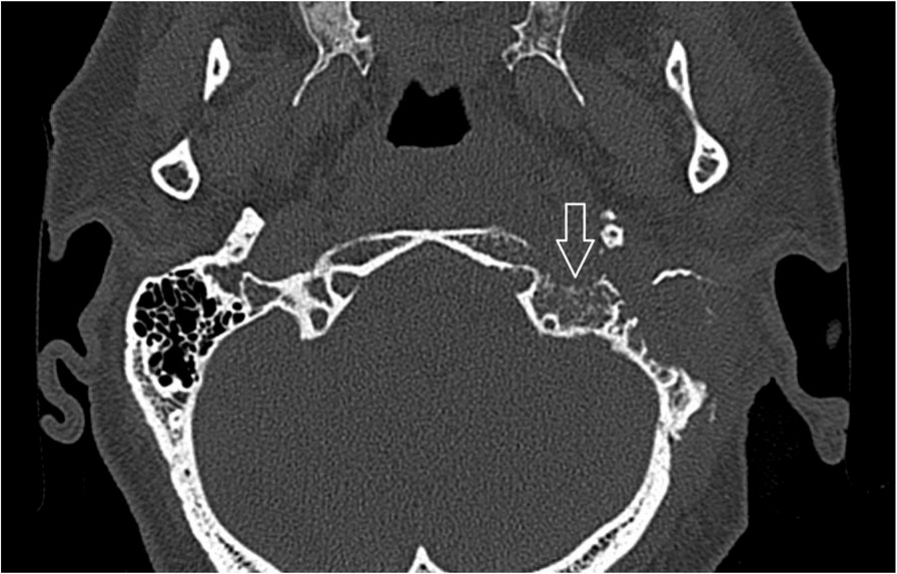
Patient No: 3, five months following mastoidectomy and antibiotic treatment: amoxicillin/clavulanic acid, gentamycin, clarithromycin and dalacin. Non-enhanced CT image (bone window) axial scan cross section of temporal and occipital bones. Osteolysis of the compact bone in the left lateral skull base represents a late sign of osteomyelitis (arrow). Minor changes of spongy bone and major changes of compact bone highlight spread of infection under the periosteum, but represents a late sign of the infection
